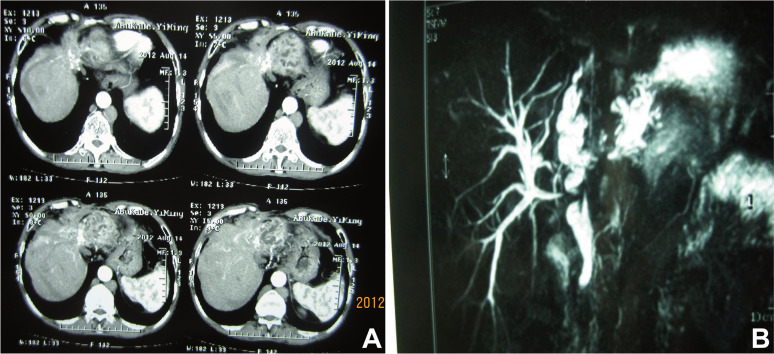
Representative imaging for type B hilar biliary duct stricture. (A) Abdominal enhanced CT demonstrating atrophy of left hepatic lobe caused by calcification of outer laminated layer, hyperplasia of right hepatic duct. (B) Abdominal MRI showing severe hilar biliary duct stricture, obvious dilatation of left hepatic duct and traction stricture of the right bile duct.
